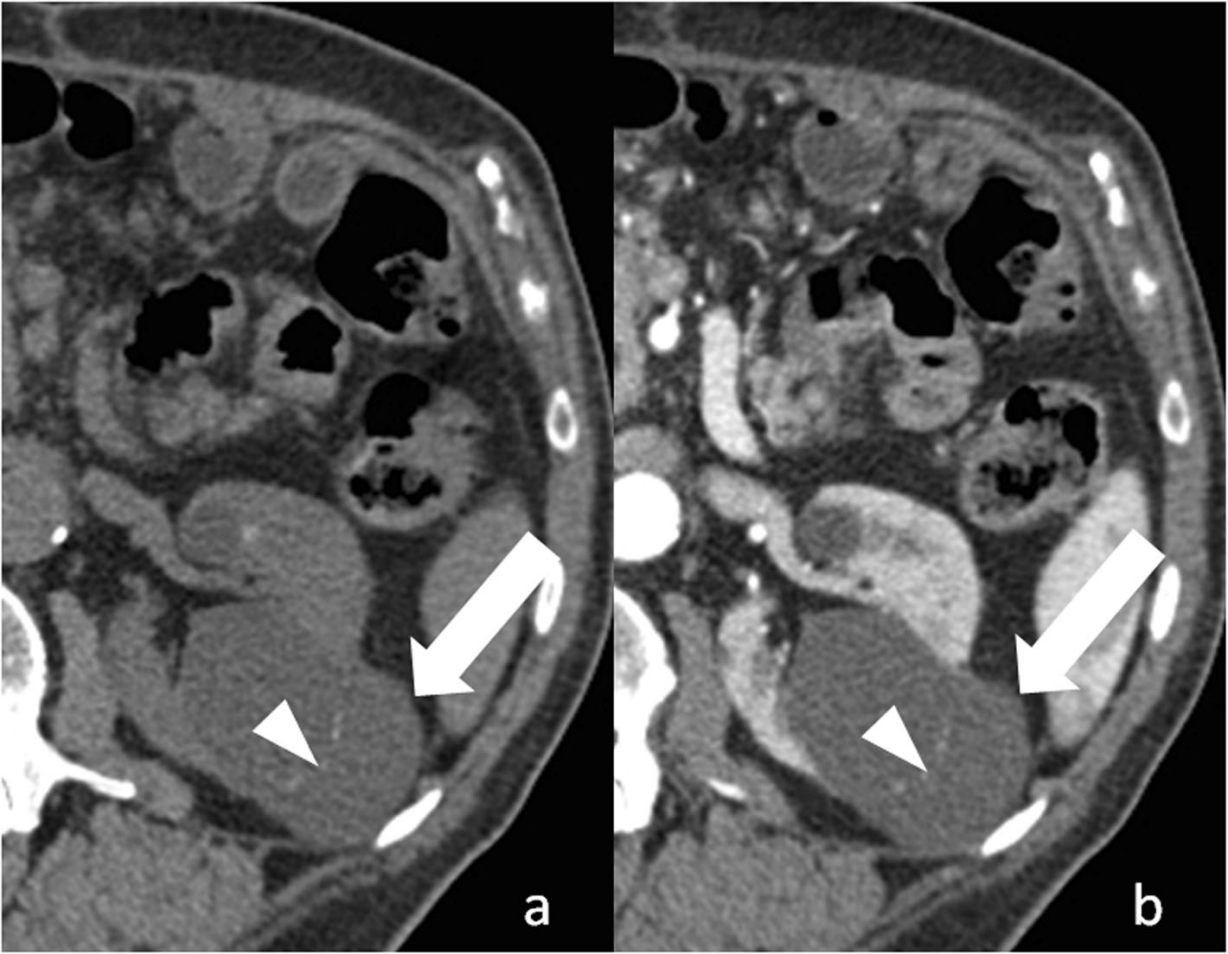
Bosniak category II renal cyst. a Axial non-enhanced CT image shows a lesion with a thin wall (arrow) and a thin septum (arrowhead), which contains fine calcifications. b Corresponding axial contrast-enhanced CT image shows enhancement of cyst wall and septum
